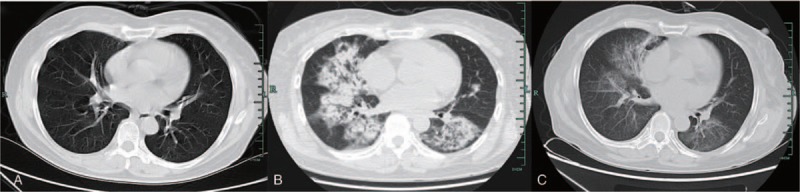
Chest computed tomography scan findings of the patient. A. One month before surgery, multiple fibro proliferative foci were seen in the lungs. B. Two days after surgery, multiple plaque-like high-density shadows were seen in both lungs, and the margins were unclear. C. Two weeks postoperatively, patchy high-density shadows were significantly absorbed compared to that of 2 days after surgery.
